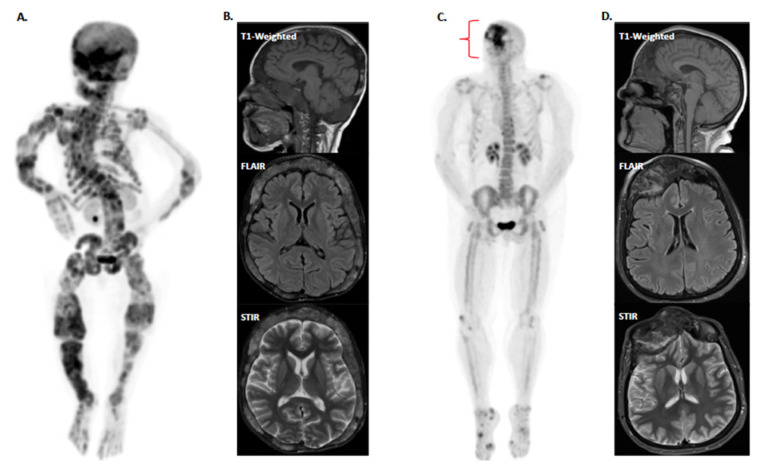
Whole-body ^18^F-NaF PET/CT and non-contrast craniofacial MRI for Patient 1 at age 23 (A,B) and Patient 2 at age 53 (C,D). (A) There is diffuse radioactive uptake in the axial and appendicular skeleton with pronounced focus in the craniofacial regions indicating polyostotic FD. (B) Craniofacial regions with diffuse FD lesions. Skull-based lesions are highlighted. (C) There is pronounced ^18^F-NaF uptake in the craniofacial region with limited uptake in the rest of the body. (D) Extensive FD affecting the nasal bones and distorting the structure of the sinus cavity. ^18^F-NaF PET = ^18^F-sodium fluoride positron emission tomography, CT = computed tomography, MRI = magnetic resonance imaging, FLAIR = fluid-attenuated inversion recovery, STIR = short tau inversion recovery.
